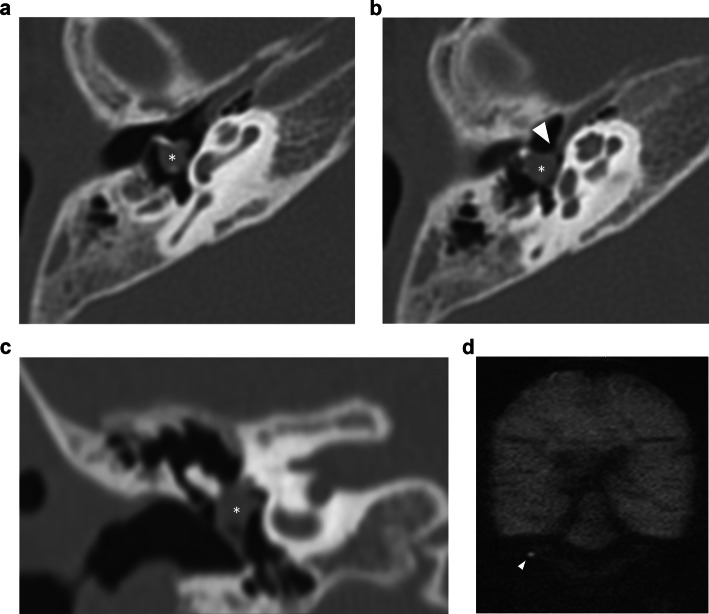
Five-year-old boy with conductive hearing loss (45 dB) on the right side. a, b Axial CT image of the right temporal bone shows a sharply delineated nodular mass lesion compatible with a CC (asterisks) growing between the malleus handle and long process of the incus and reaching the tensor tympani tendon superiorly (arrowhead). c Normal appearing scutum and Prussak’s space on this coronal reformatted CT image of the right temporal bone. Note lysis of the lenticular process of the incus which should be visible on this slice, corresponding to a Potsic stage 3. d Lesion correlating with a hyperintense focus on non-EP DWI sequence, confirming the diagnosis of a CC
